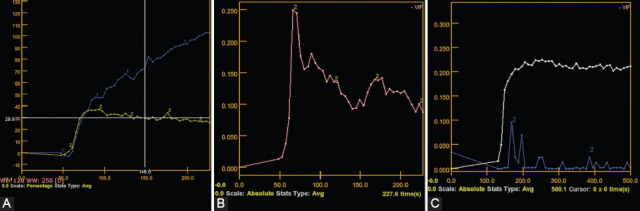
Dynamic contrast-enhanced magnetic resonance imaging. (A) Pleomorphic adenoma (curve type A – curve number 1 on the presented image); (B) Warthin tumor (curve type B); and (C) malignant tumor (curve type C).
